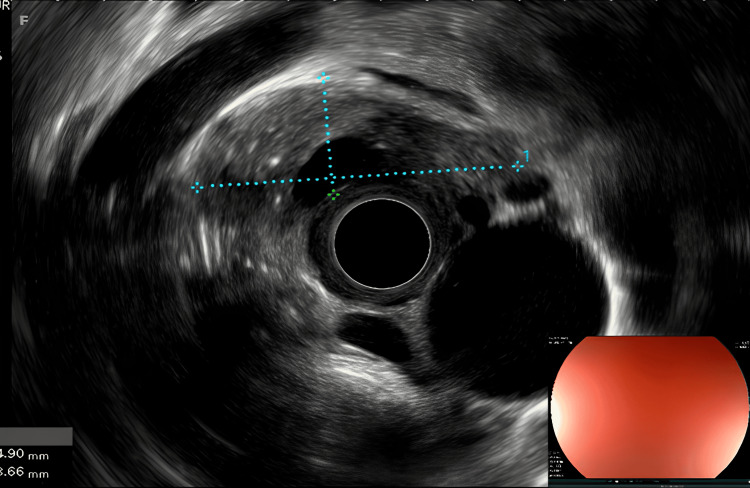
Endoscopic ultrasound of the ulcer showing hemicircular thickening of the esophageal wall.
